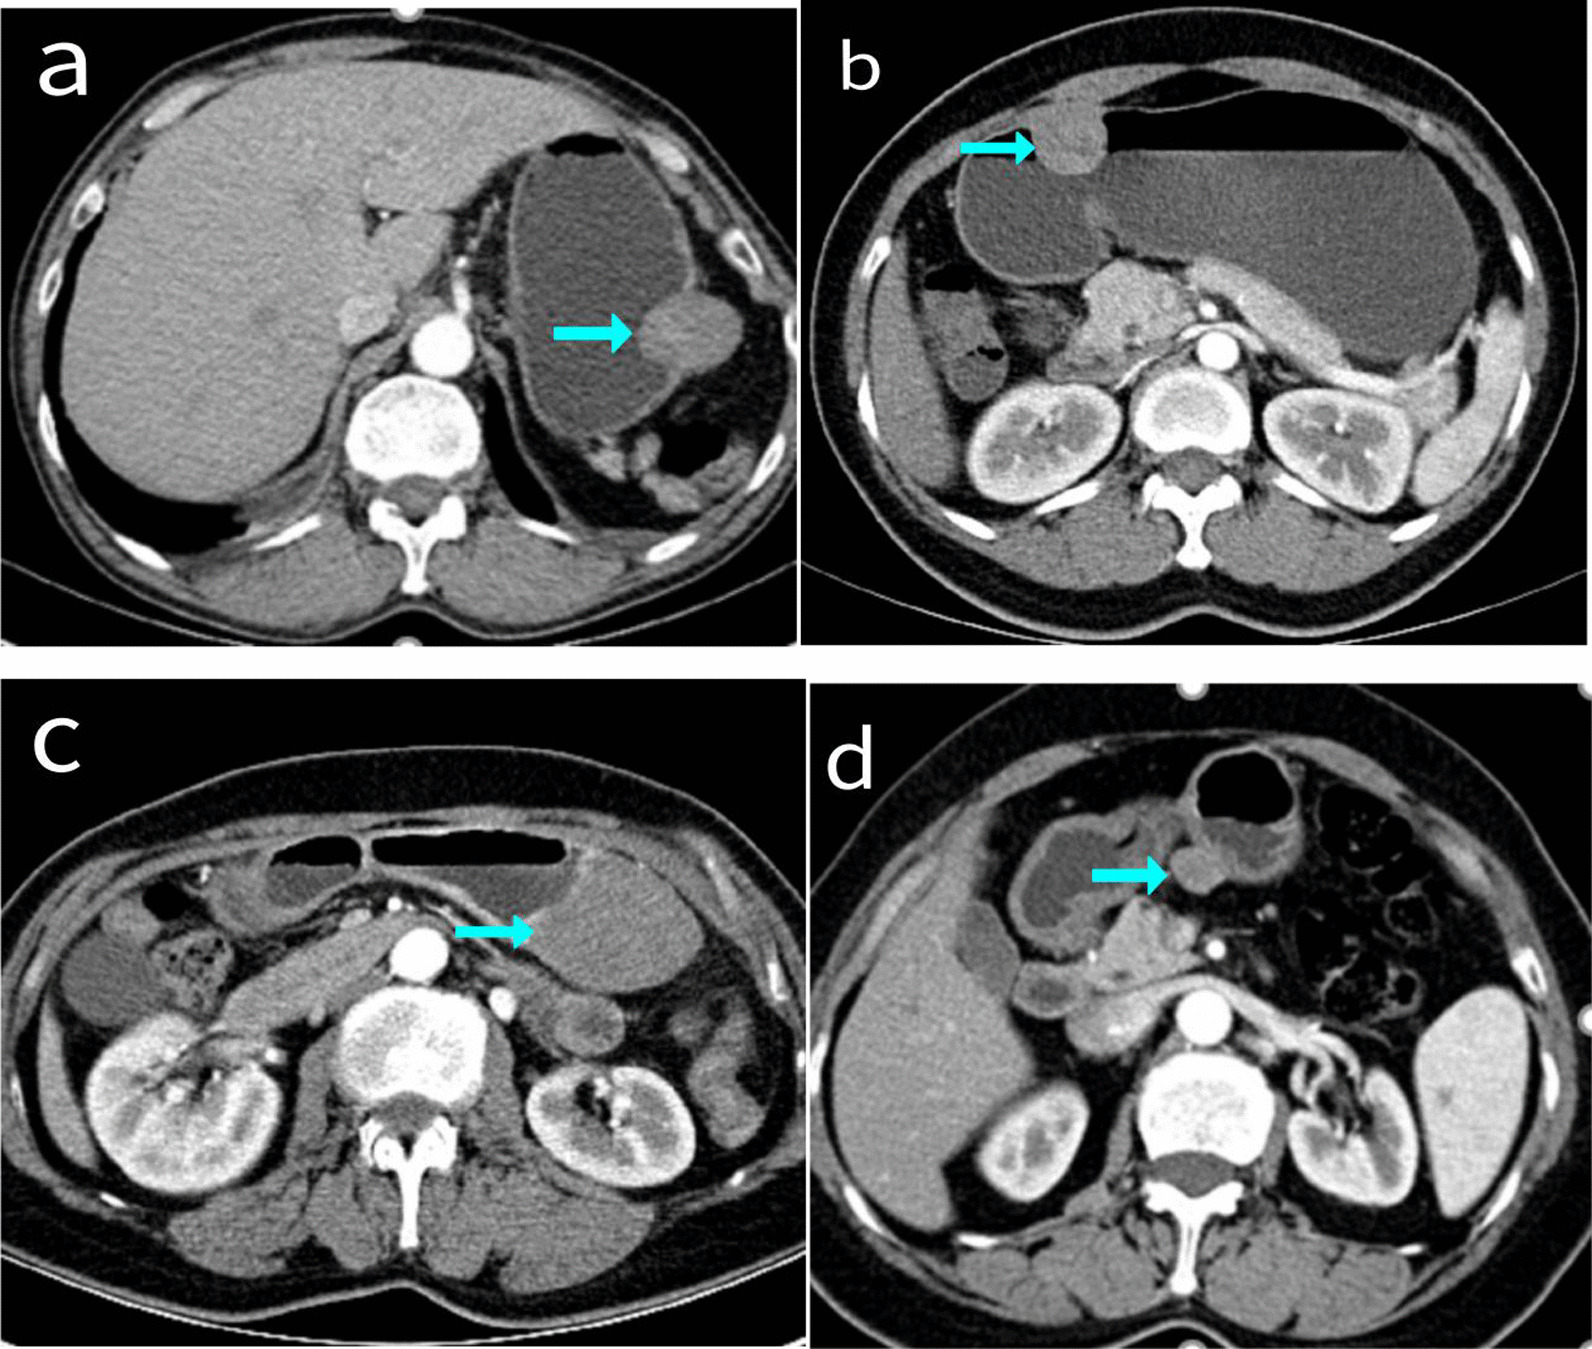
Enhanced CT images of four cases of gastric schwannoma
